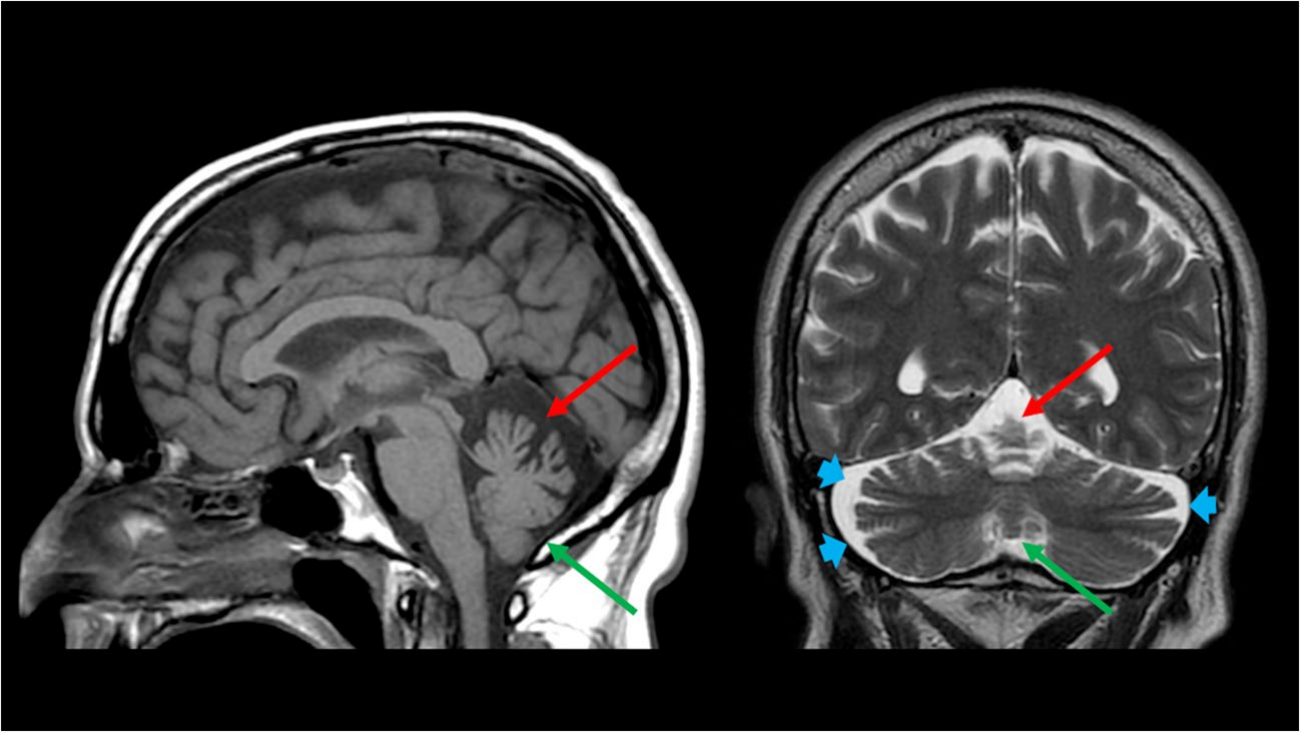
A pattern of “pure” cerebellar atrophy. Sagittal T1-weighted (*left*) and coronal T2-weighted (*right*) images of a 54-year-old male Spinocerebellar Ataxia Type 6 patient showing a pattern of “pure” cerebellar atrophy, with a concomitant involvement of the superior vermis (*red arrows*), and the cerebellar hemispheres (depicted by the enlargement of the cerebellar fissures with corresponding increase in CSF spaces—*blue arrowheads*), and a less significant although present volume loss of the inferior vermis (*green arrows*), with a relative sparing of the remaining brain structures
